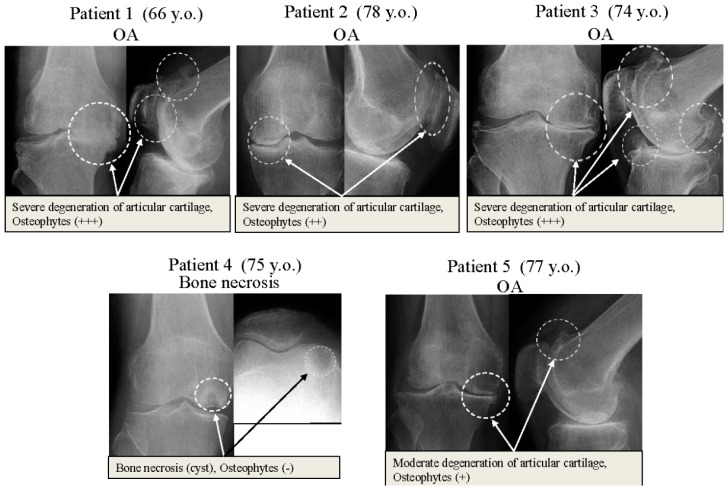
X-ray images of knee joints of donor patients. Patient 1; female, 66 years of age, Patient 2; female, 78 years of age, Patient 3; male, 74 years of age, Patient 4; female, 75 years of age, Patient 5; female, 77 years of age. Patient 1, 2, 3 had severe knee OA with severe cartilage degeneration and osteophyte formation. Patient 4 had idiopathic osteonecrosis of femoral condyle. Patient 5 had moderate knee OA with cartilage degeneration and osteophyte formation. OA; osteoarthritis, y.o.; years old.
